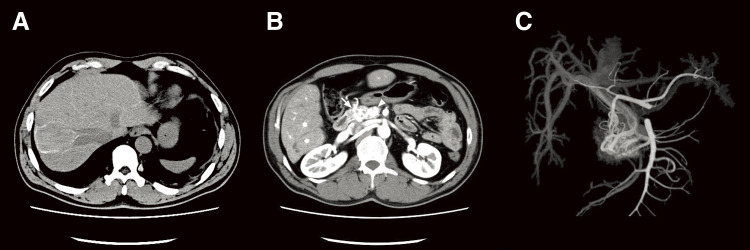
Findings of computed tomography (CT). (A) Non-enhanced CT showed the presence of intrahepatic reticulated calcification, indicating the presence of *Schistosomiasis japonica*. (B) Contrast-enhanced CT demonstrated the enhancement of the pancreatic head (arrow) and main portal vein (arrowhead) in the late arterial phase. (C) Maximum intensity projection images revealed blood flow from the gastroduodenal artery and superior mesenteric artery to the main portal vein.
